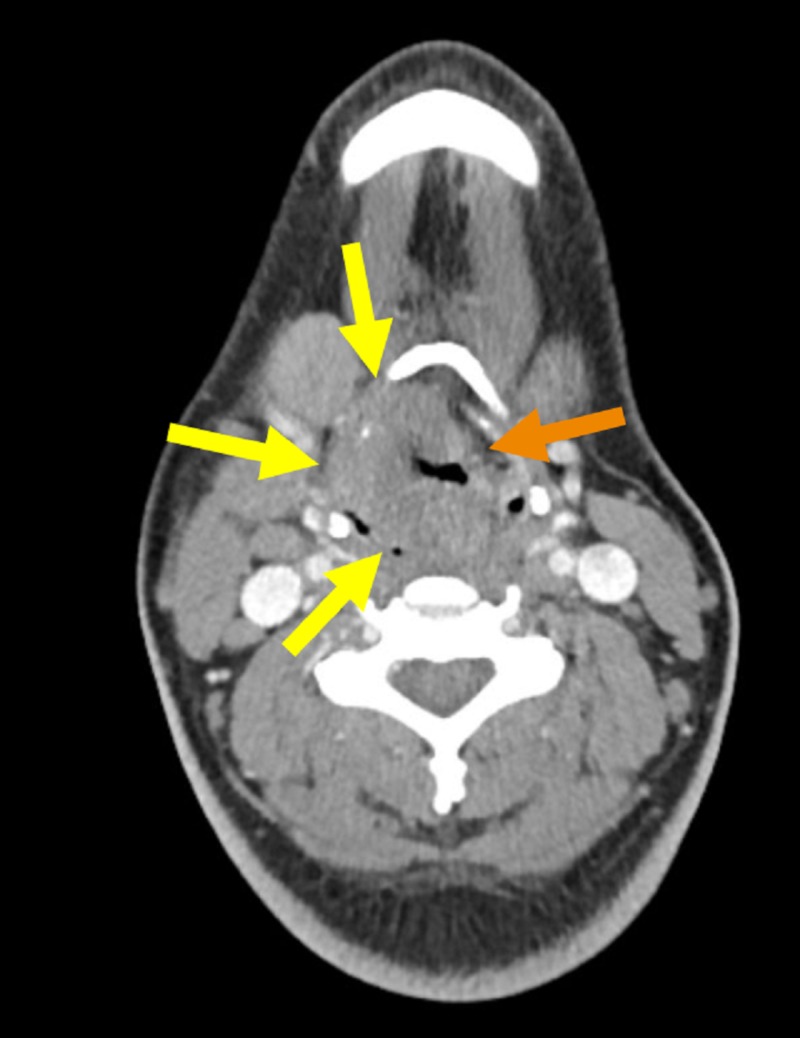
Axial contrast-enhanced computed tomography (CT) image of the neck. Yellow arrows indicate the mass (located within the right hypopharynx). Orange arrow signals the normal contralateral left hypopharyngeal wall.
